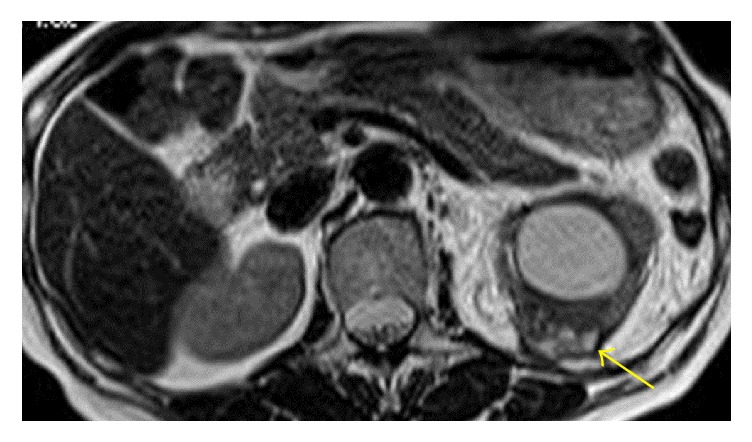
MRI: the tumor contained small cysts (arrow).
